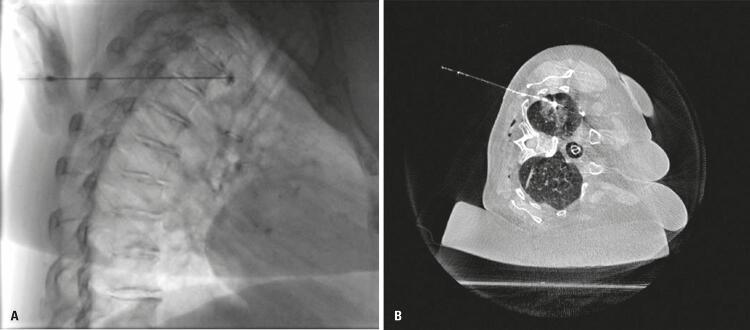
(A) Lipiodol® injection in pulmonary parenchyma guided by cone beam computed tomography and fluoroscopy; (B) Final cone-beam computed tomography showing appropriate Lipiodol® and hookwire location
